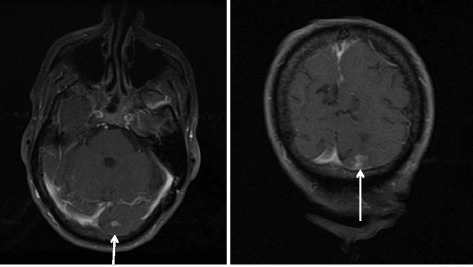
Coronal T1-weighted postcontrast magnetic resonance images demonstrating a subcortical density (*arrows*) in the subcortical region of the inferior left occipital lobe
